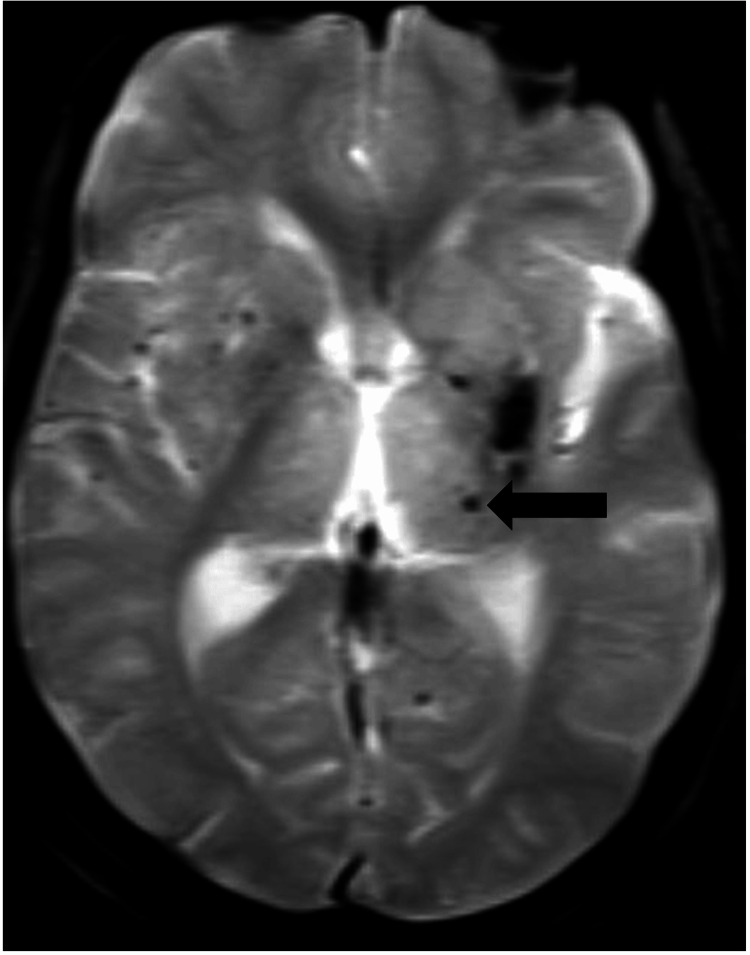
Blooming noted in MRI, suggestive of microhemorrhages in the thalamus (as shown by the black arrow).
